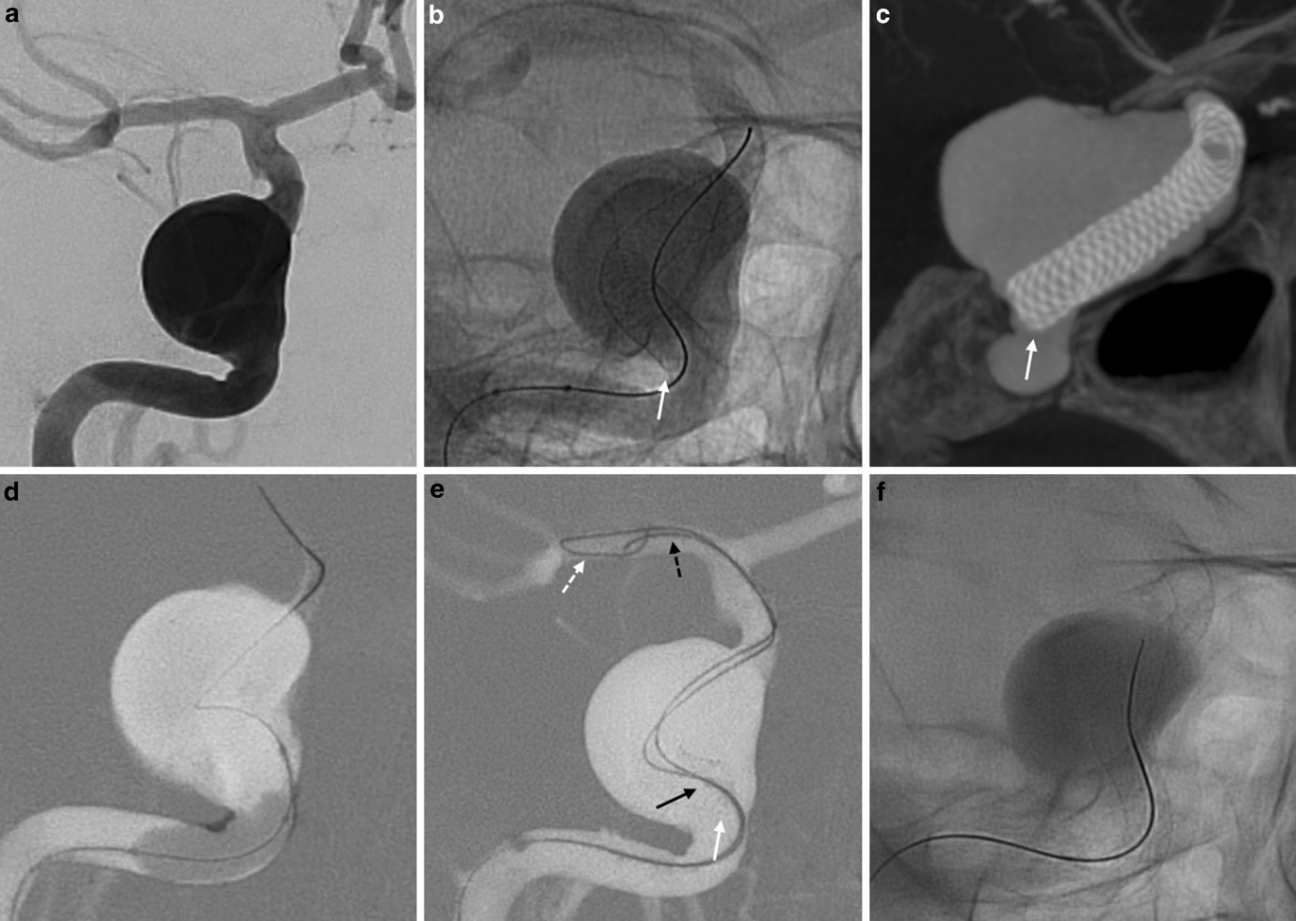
Female patient presenting with right-sided ophthalmoplegia. The CT angiogram (CTA, not shown), and later an intraprocedural prestenting angiogram (a), demonstrated a large ipsilateral cavernous carotid aneurysm. b Treatment was pursued using a 4 mm × 50 mm Stryker Surpass Streamline flow diverting stent delivered through a Stryker XT-27 microcatheter. The proximal end of the FD (*white arrow*) landing a little too short. c Cone beam CT demonstrated poor wall apposition of the proximal end of the FD (*white arrow*). (d) Angioplasty of the proximal FD was performed to improve wall apposition. e This resulted in foreshortening and distal migration of the proximal end of the FD (*white arrow*) upon balloon removal, with prolapse of the proximal stent into the aneurysm. Access into and through the stent lumen was regained using the buddy-wire technique with a Synchro 0.014in microwire (*white dashed arrow*) and X‑pedion 0.010in. guidewire (*black dashed arrow*) allowing distal navigation of the XT-27 microcatheter (tip of the catheter, *black arrow*). f A second Surpass Streamline FD was deployed telescopically covering the proximal end of the aneurysm and improving wall apposition of the first FD
